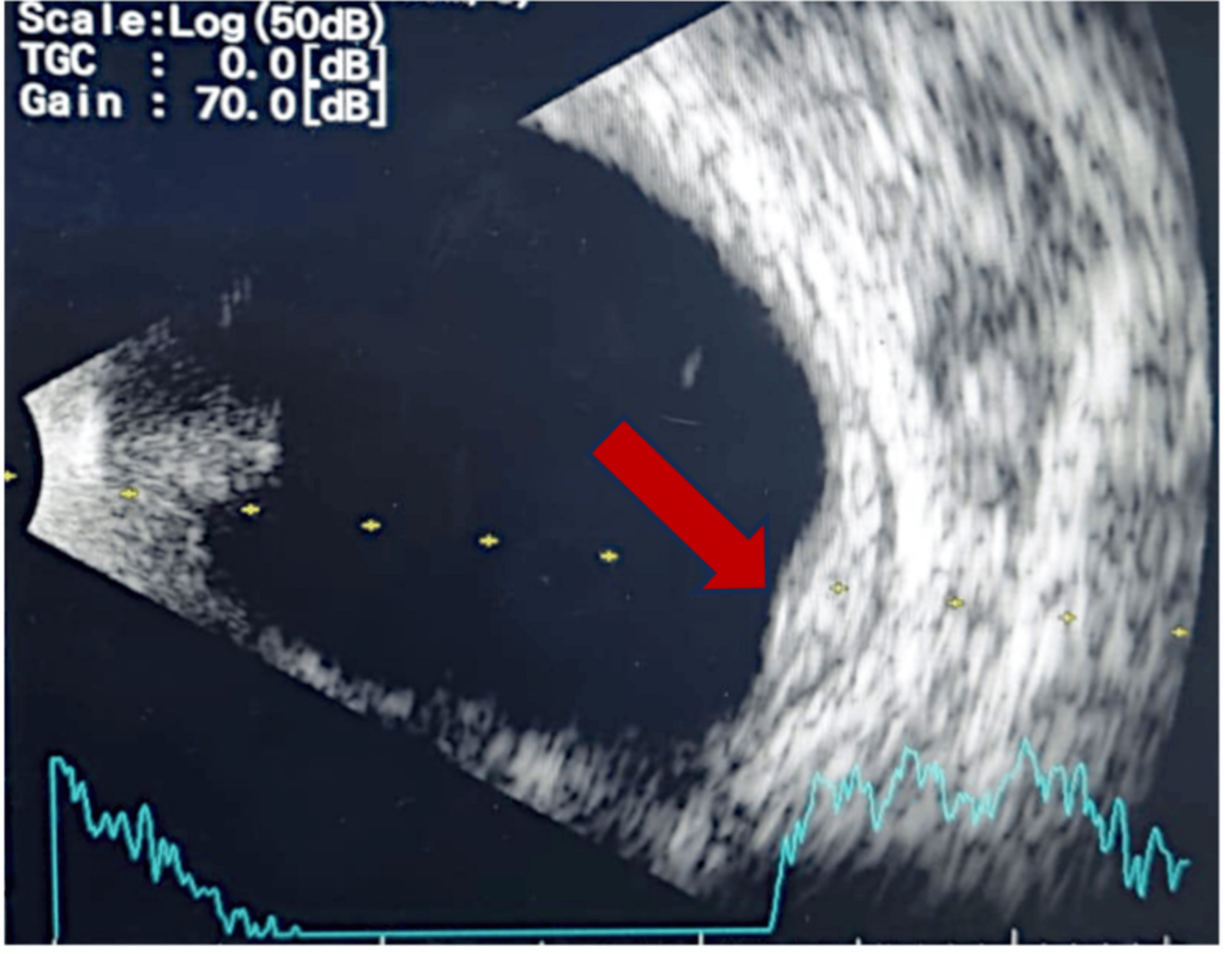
B-scan ultrasonography showing a thickened choroid with fluid in the subtenon's space (red arrow)
